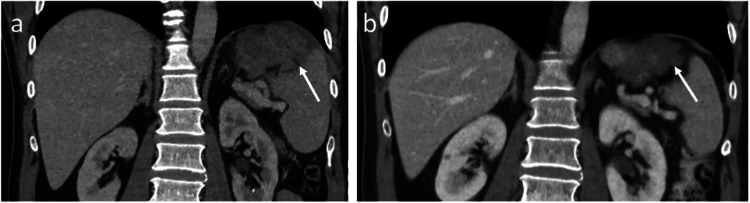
Coronal portal venous phase CE-CT images of a patient diagnosed with a high-risk gastric GIST (white arrows). a) On baseline CE-CT the tumour seemed adherent to the spleen and the left hemidiaphragm, which would suggest the need for a splenectomy. b) The preoperative CE-CT, showed a reduction in tumour size after 11.7 months of neoadjuvant TKI treatment (imatinib). The tumour no longer appeared adherent the spleen and left hemidiaphragm. Surgical benefit was gained in this patient, as TKI therapy facilitated spleen-sparing surgery.
